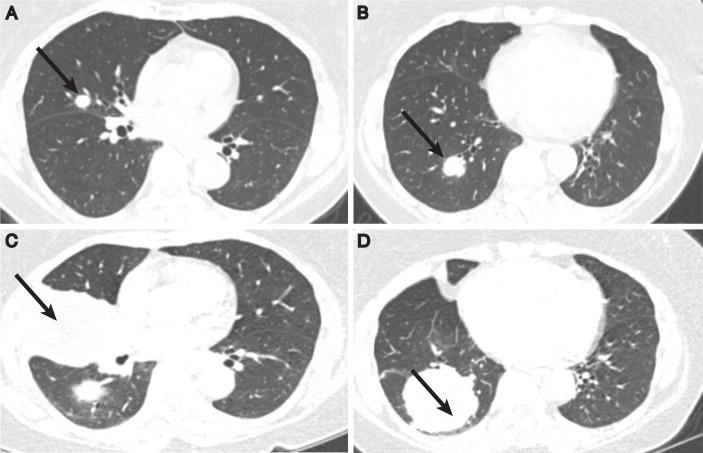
Chest CT imaging demonstrating pulmonary masses at the time of diagnosis in the right middle lobe (A) and the right lower lobe (B). Despite platinum doublet chemotherapy and immunotherapy, imaging showed significant enlargement of the right middle lobe (C) and right lower lobe (D) lesions prior to her bilobectomy.
